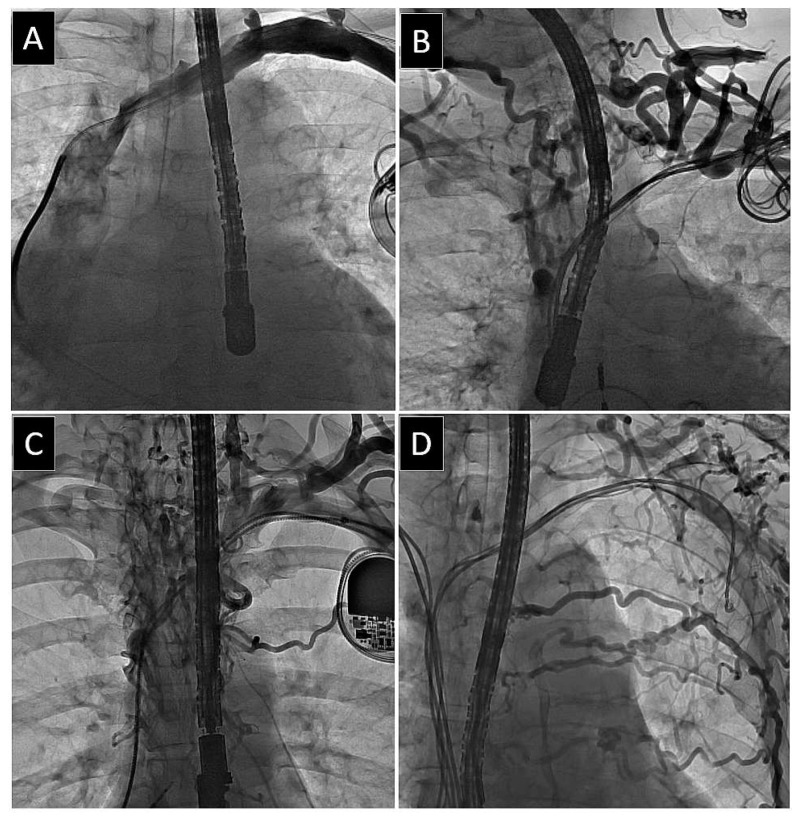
Examples of lead-related obstruction of the major thoracic veins. Leads on the left side of the chest. A transesophageal echocardiographic probe can be seen. (A) Severe stenosis isolated to one vein (brachiocephalic). (B) Two veins affected. Occlusion of subclavian and brachiocephalic veins. (C) Three veins affected. Stenosis of subclavian vein, occlusion of brachiocephalic vein, and severe stenosis of superior vena cava. (D) Four veins affected. Occlusion of axillary, subclavian, and brachiocephalic veins, and severe stenosis of superior vena cava. Well-developed collateral circulation through neck and thoracic (B–D) veins.
